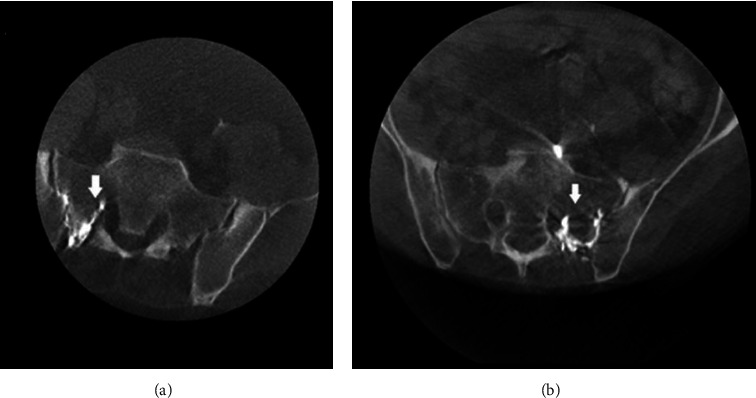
(a, b) 3D-CBCT images of two patients who received sacroiliac joint injections (the arrow indicates contrast spread from sacroiliac joint into posterior S1 neuroforamen).
